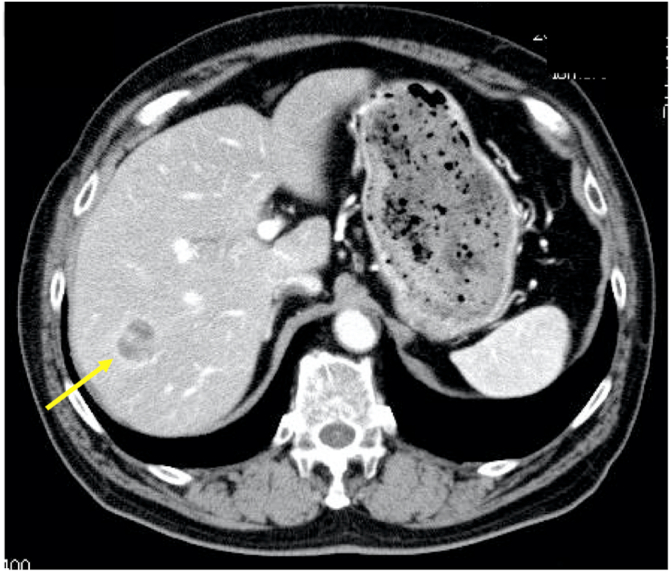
Preoperative CT A well-defined low density tumor with ring enhancement measuring 2.5 cm in diameter is seen in hepatic segment 6.
